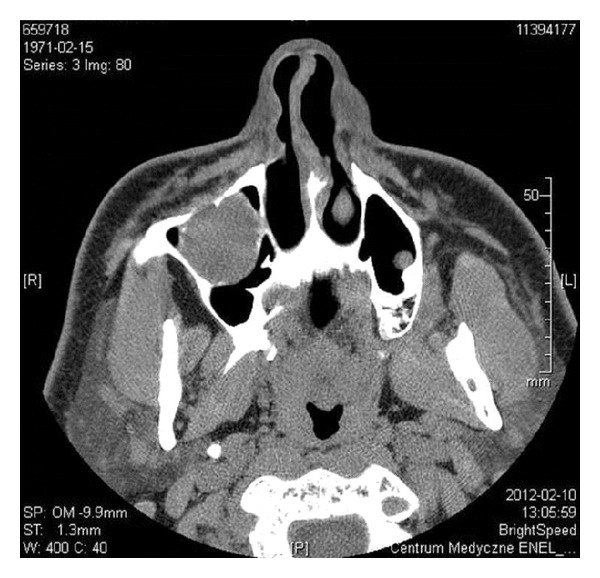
CT: 3 cm diameter, oval hypodense tumor in right maxillary sinus.
